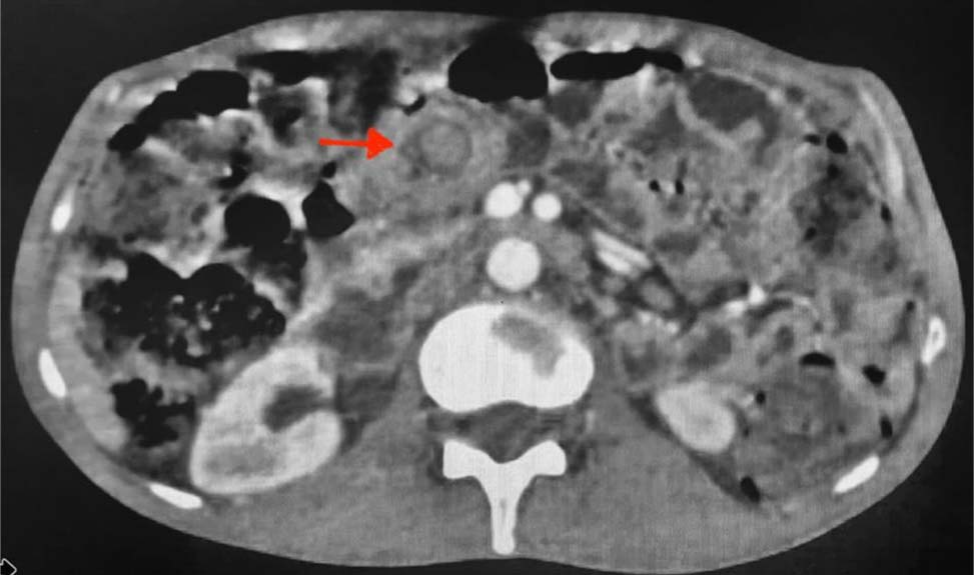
Positron emission tomography-computed tomography axial image showing heterogeneously enhancing soft tissue lesion in the proximal jejunal loop with associated intussusception (target sign).
